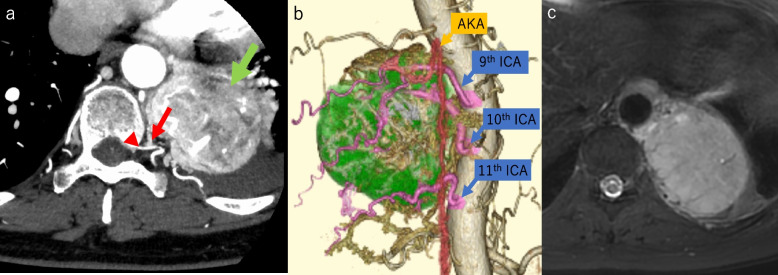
a Computed tomography revealed a large tumor (8.1 × 7.6 × 6.4) in the left posterior thorax that was in contact with the chest wall, mediastinum, and descending aorta. The T9th ICA (arrow) flowed into both the tumor (green arrow) and the AKA (arrowheads). b 3D‐CT image reconstruction showed the tumor occupying the left thorax (green) and the tumor‐feeding artery (the T9–T11th ICAs are colored in pink). The small arrow indicates the T9th ICA, and the arrowhead indicates the AKA. c T2-weighted MRI revealed a spherical tumor with high signal intensity
